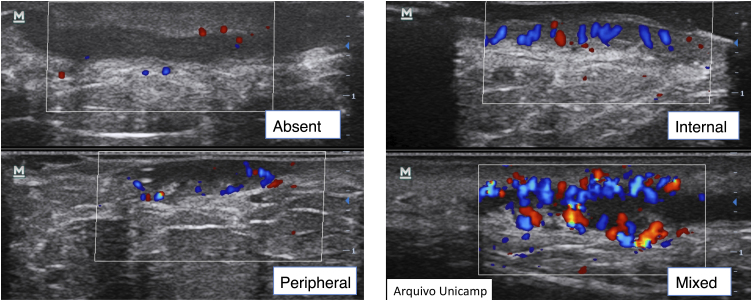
Type of vascularization on Doppler.
